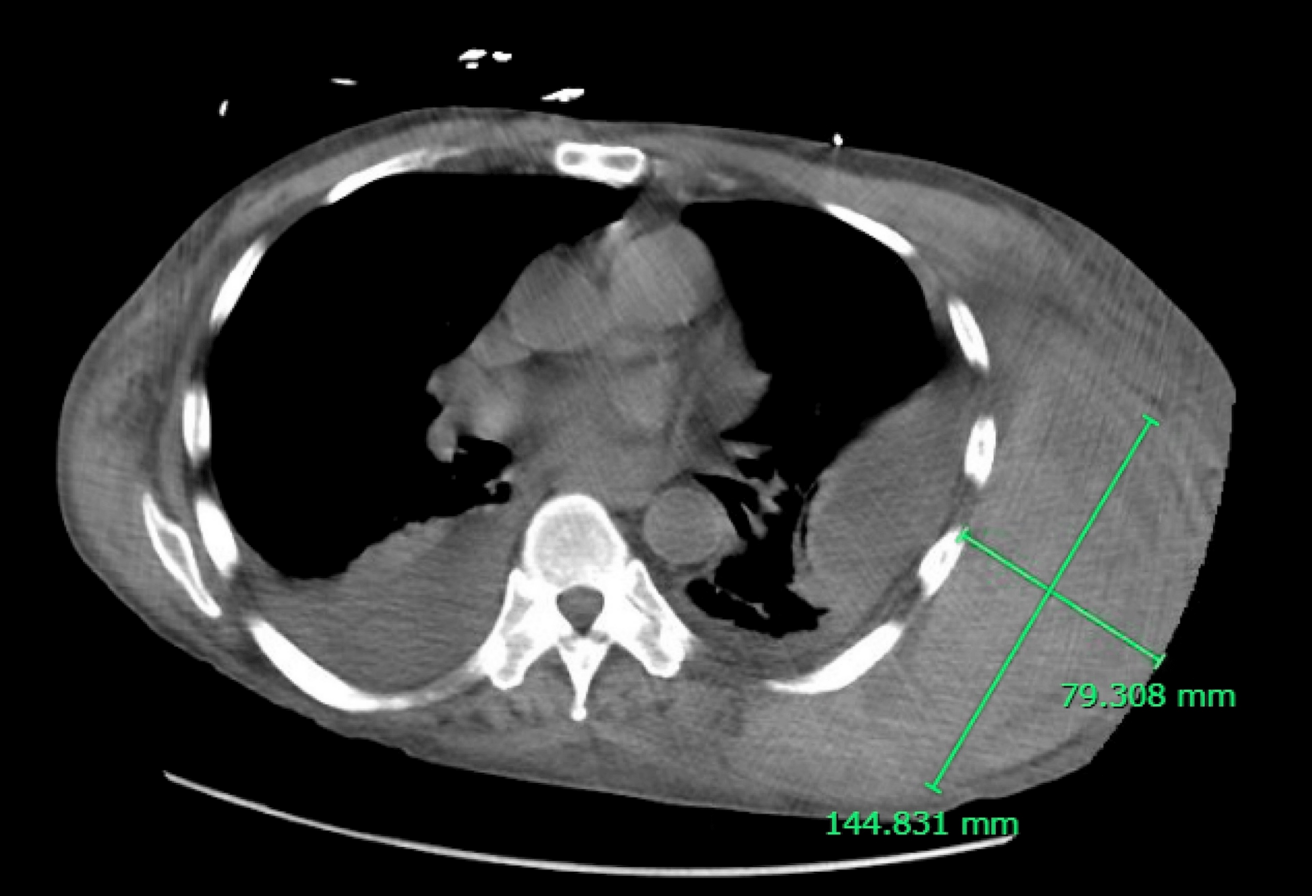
Axial computed tomography of the chest demonstrating a large left chest wall hematoma.
